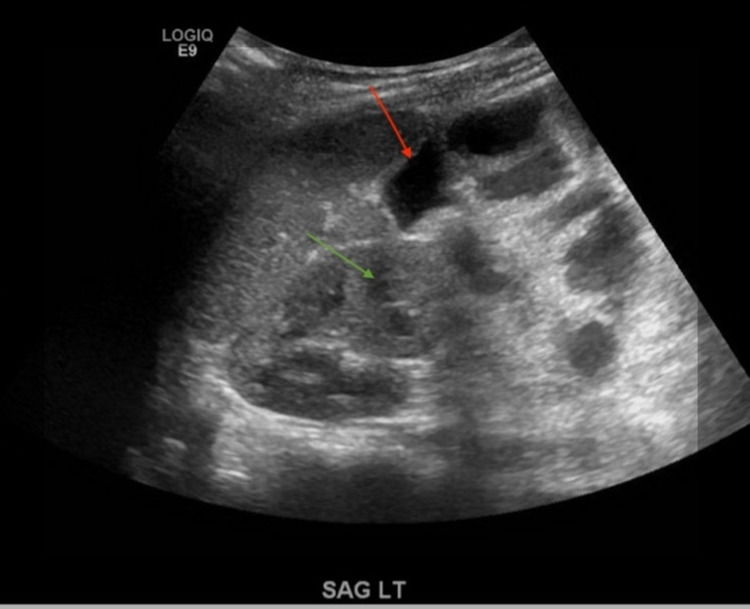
Abdominal ultrasound of right kidney with hydronephrosis(green arrow) and prominent bowel loops(red arrow).
